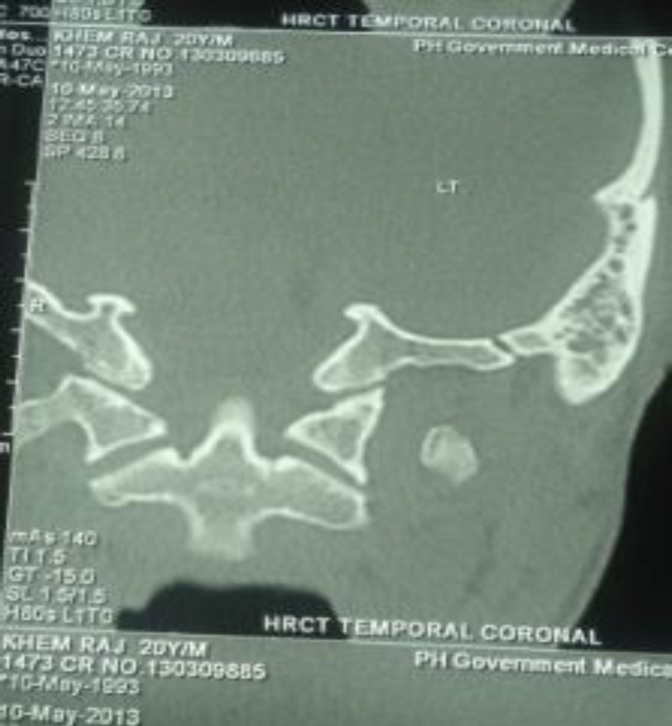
HRCT temporal bone showing mastoid destruction with haziness of cells
